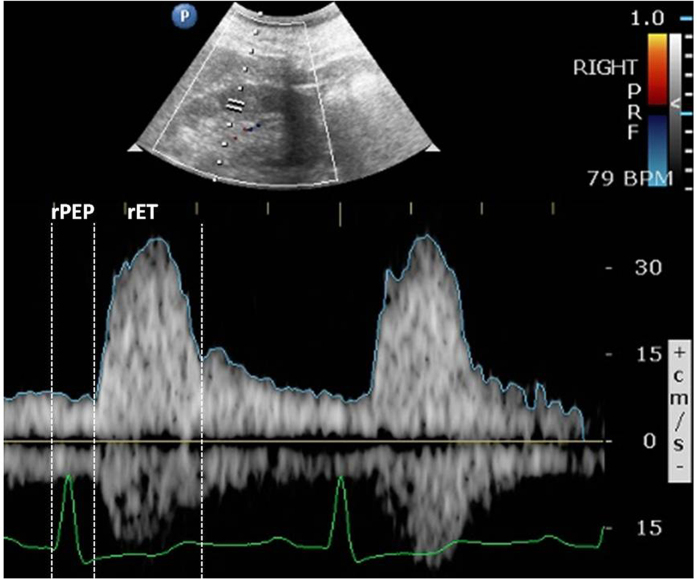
The renal pre-ejection period (rPEP) was measured from the onset of the QRS complex to the foot of the renal pulse Doppler waveform. The renal ejection time (rET) was measured from the foot to the dicrotic notch of the renal pulse Doppler waveform.
